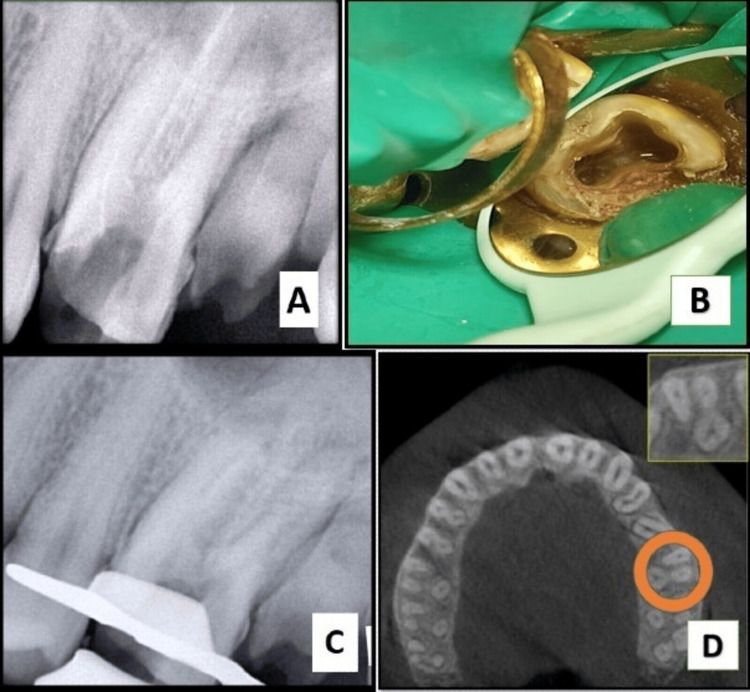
(A) Preoperative radiograph showing inadequate obturation and loss of coronal restoration. (B) Redefining the access cavity. (C) Gutta-percha removal. (D) Cone-beam computed tomography image showing a single canal in the mesiobuccal root.
